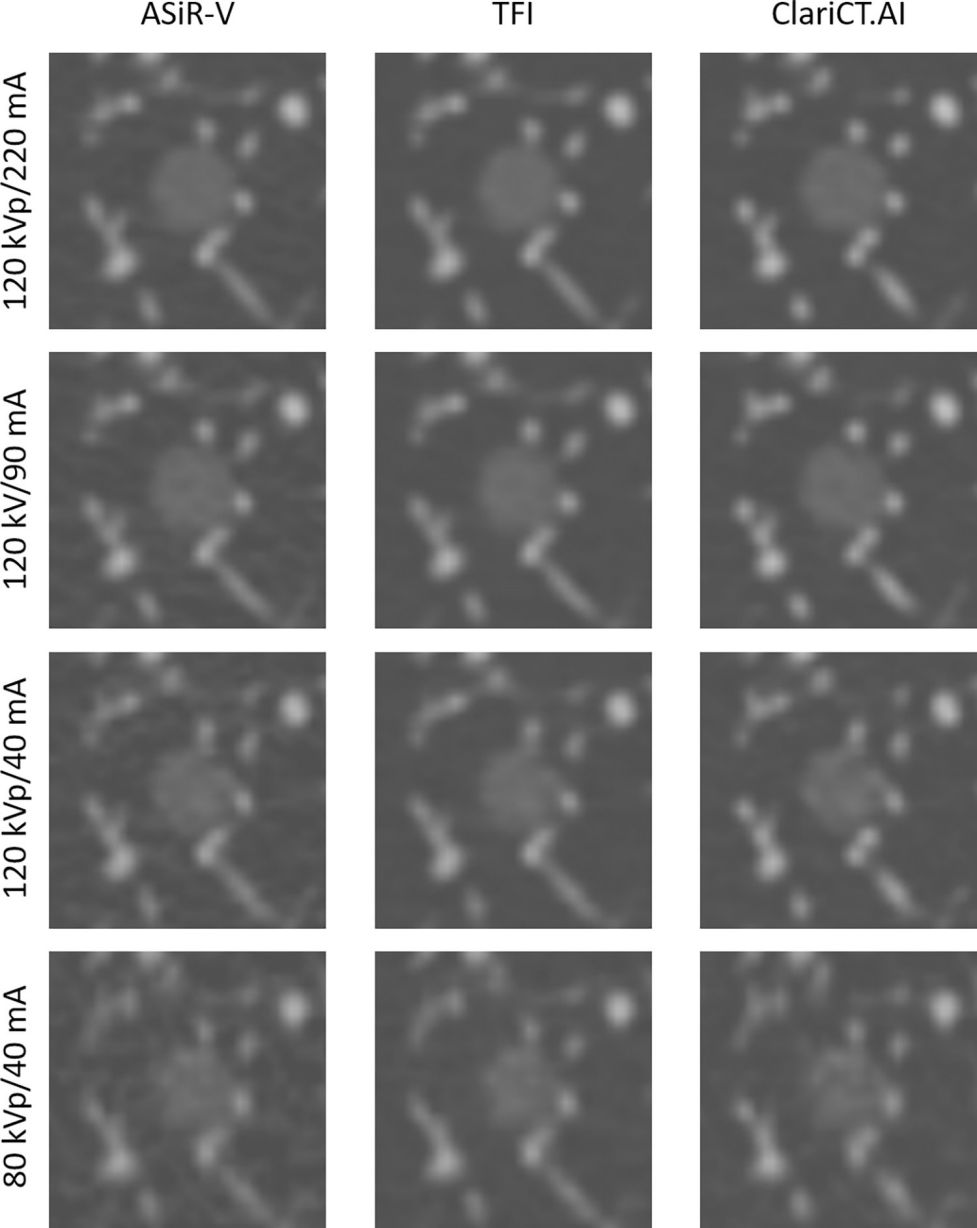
CT images of GGNs at different radiation dose settings. Images of GGNs (8 mm) with an attenuation of −800 HU at four radiation dose settings reconstructed with ASiR-V, TFI, and ClariCT.AI.
